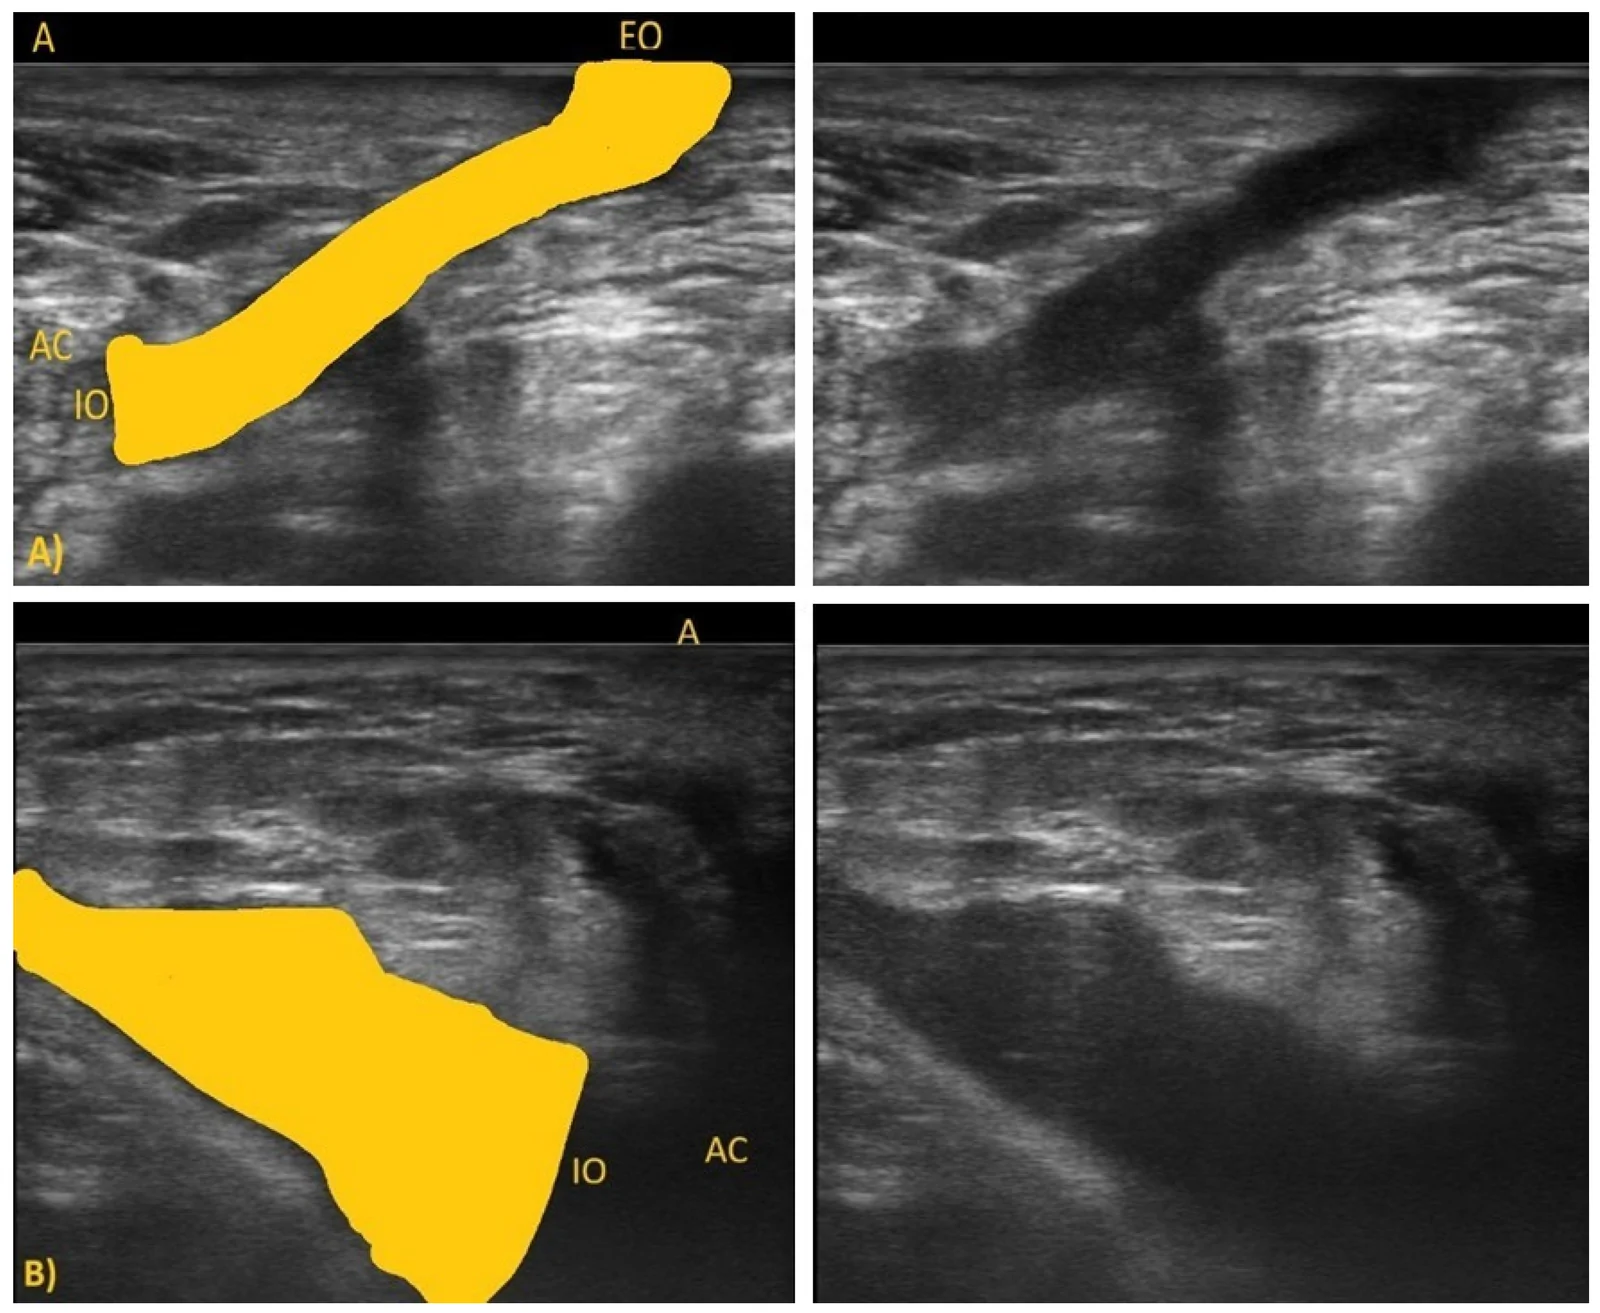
Ultrasound images obtained with a superficial linear probe (7.5 MHz) shows a complex bilateral perianal fistula with image (A) a right fistulous tract measuring 4 cm in length, with an obvious external opening (EO) and a single internal opening (IO) into the anal canal (AC) located 15 mm away from the anal verge (A), and image (B) Another left-sided fistulous tract with an IO into the AC located 34 mm away from the anal verge (A) with no EO. The left images show the tract of the fistula (thick yellow line), EO, and IO. The right images are the corresponding unannotated scans.
